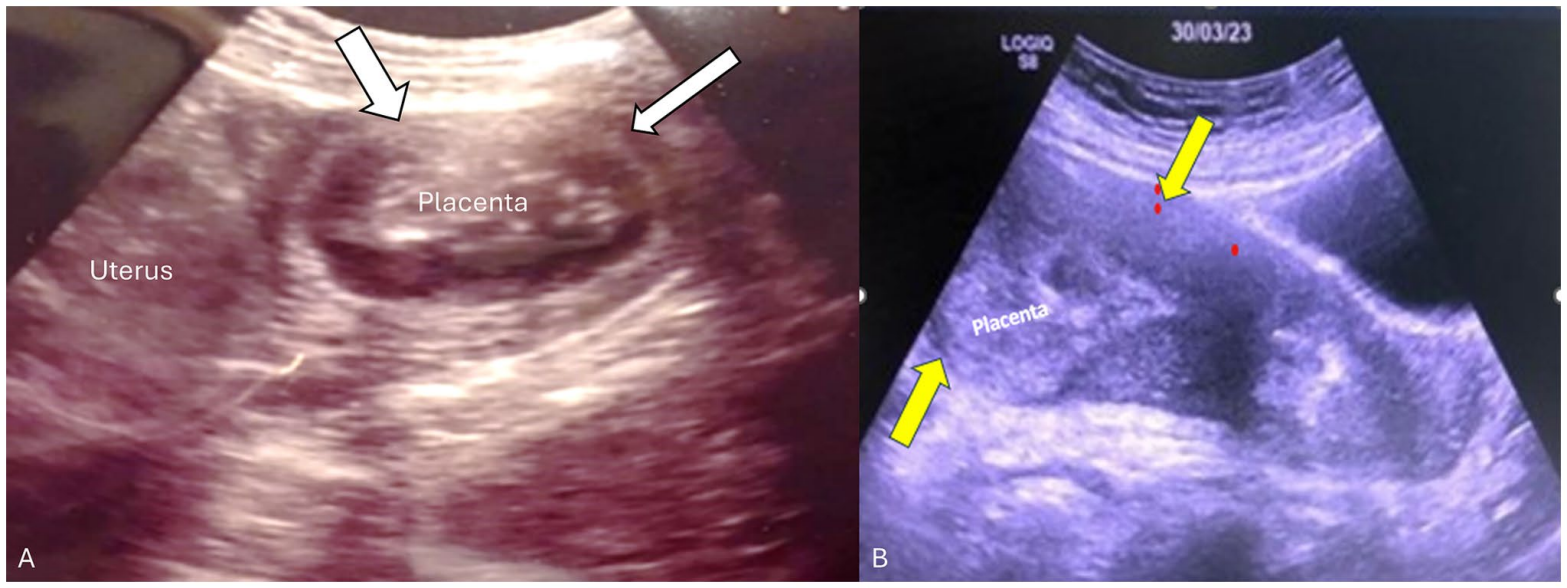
Transabdominal ultrasound of the placenta and uterus in both patients. (A) Transverse view of the uterus showing the placenta in the left cornua 14 weeks postpartum. Myometrial thinning was seen in the fundal portion adjacent to the placenta consistent with placenta accreta/adherent (white arrows). (B) Sagittal view of the uterus showing retained fundal placenta. Yellow arrows demonstrate a thick contracted anterior-wall myometrium whereas the posterior uterine wall is very thin (non-detached partial placenta adherent).
